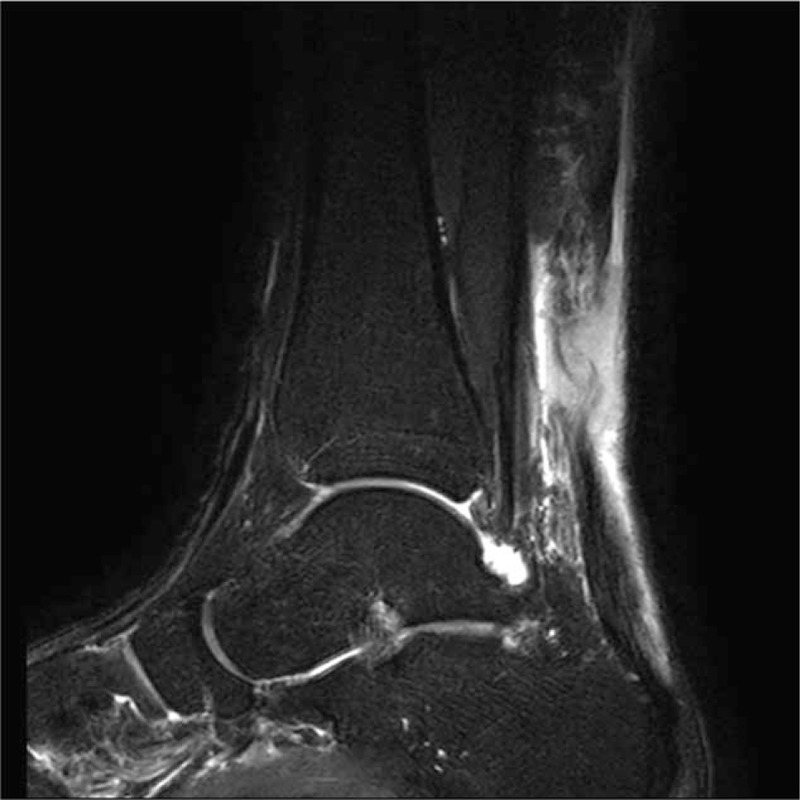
An ankle MRI image showing rupture of the Achilles tendon. On the fat-suppressed T2-weighted sagittal image, there is disruption of the Achilles tendon with a gap of about 4 cm at the upper 6 cm of the Achilles tendon calcaneal insertion.
